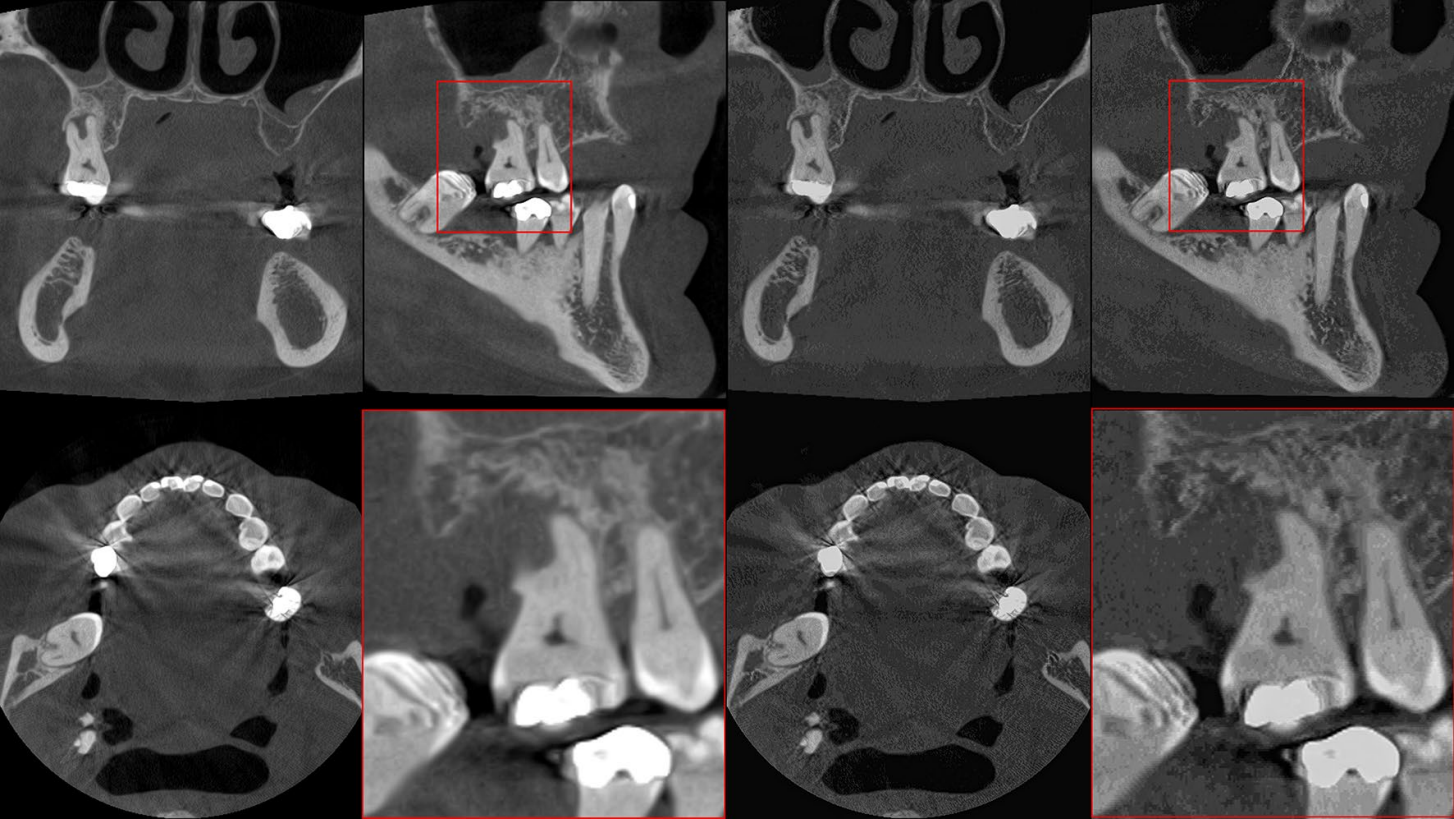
Examples of maxillofacial cone-beam CT images (left) and corresponding super-resolution predictions (right). Predictions are shown from the structure model, without averaging the orthogonal planes
